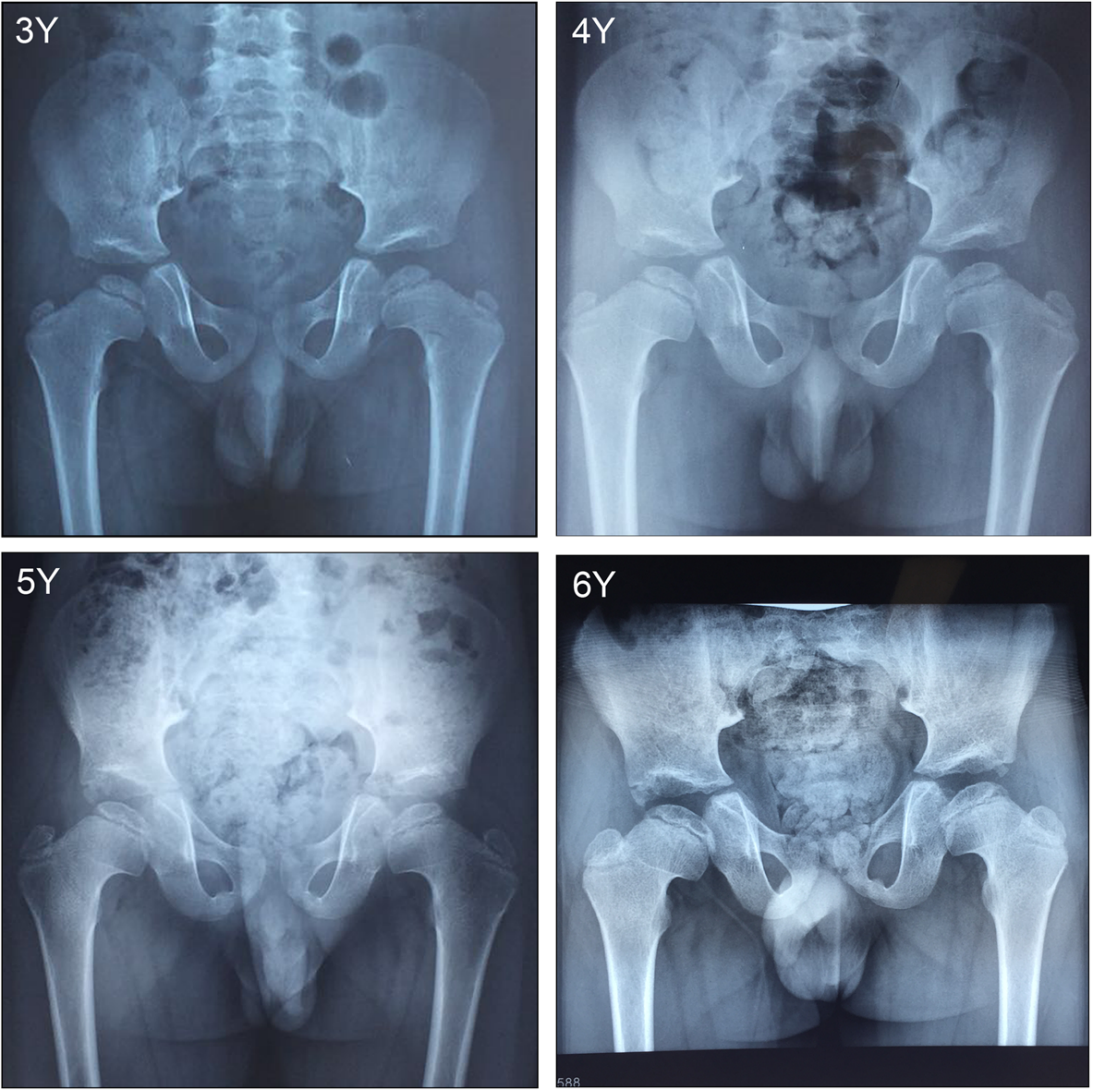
Hip radiographs of patient 2 during the ages from 3 to 6 years. Roentgenologic bone survey showed hip dysplasia with the following abnormalities: both femoral necks were short with flattened heads, acetabulum dysplasia, and secondary ossification center of femur dysplasia
